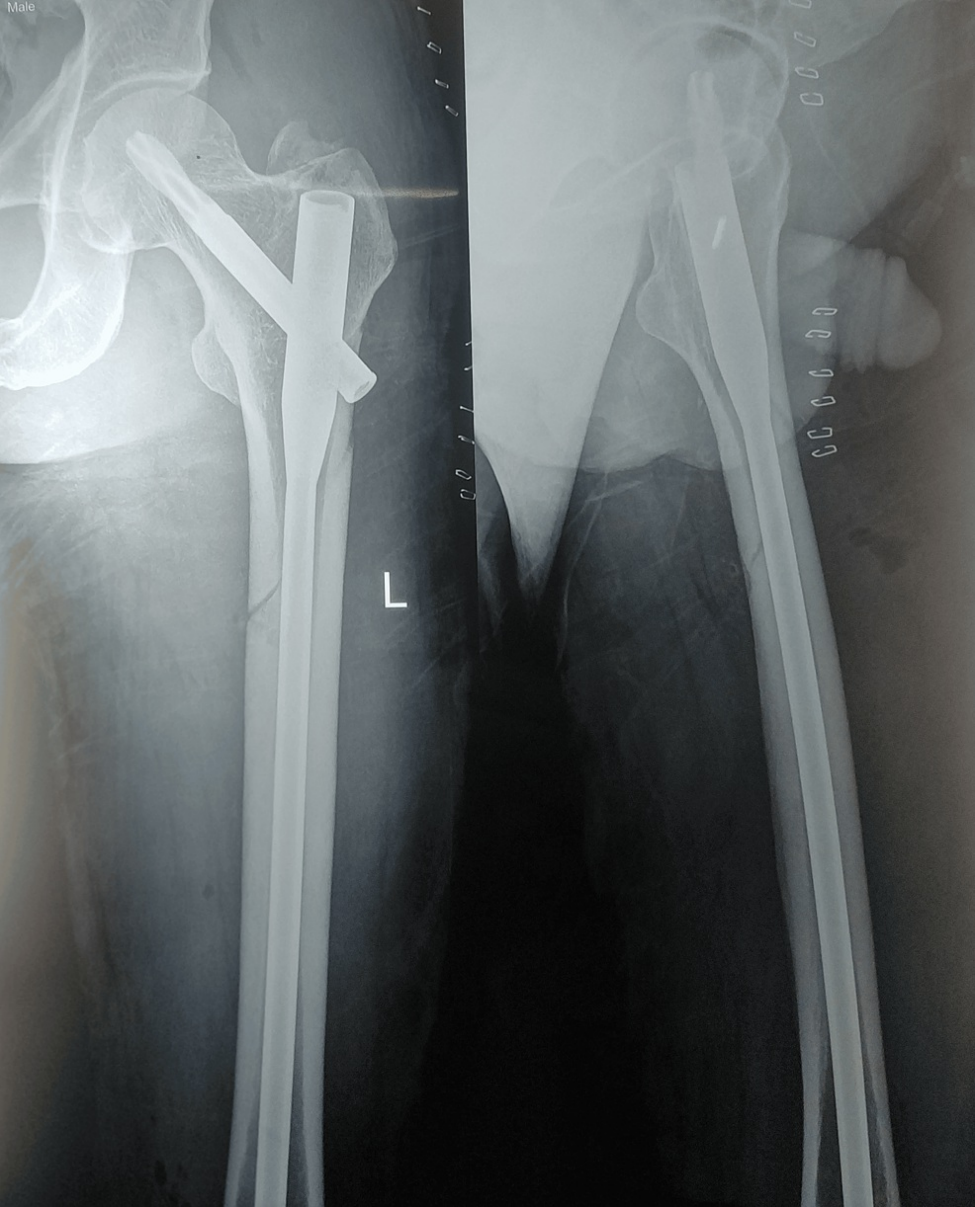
Post-operative X-ray (AP view) showing subtrochanteric fracture fixed with long PFNA-2 PFNA-2, proximal femoral nail antirotation-2; AP, anteroposterior
